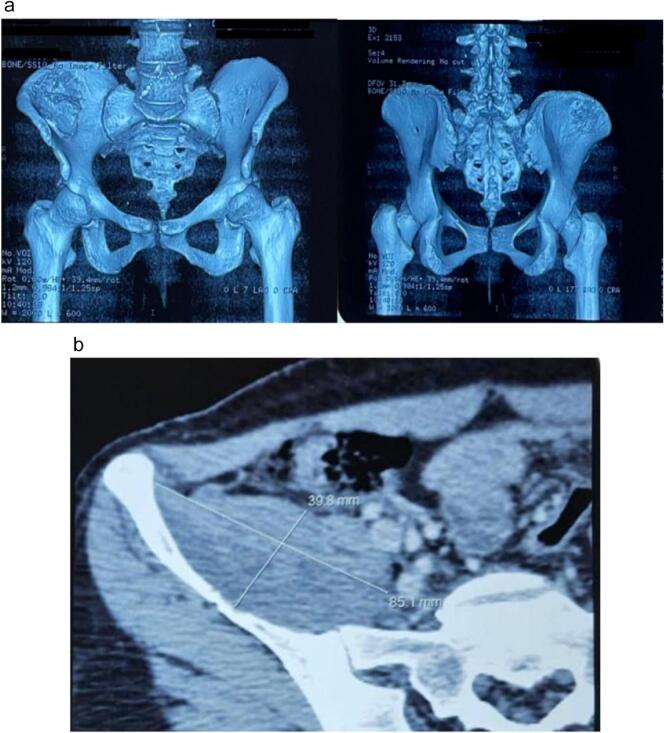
Three-dimensional CT scan of the lesion b: CT scan image showing the size of the tumor.
